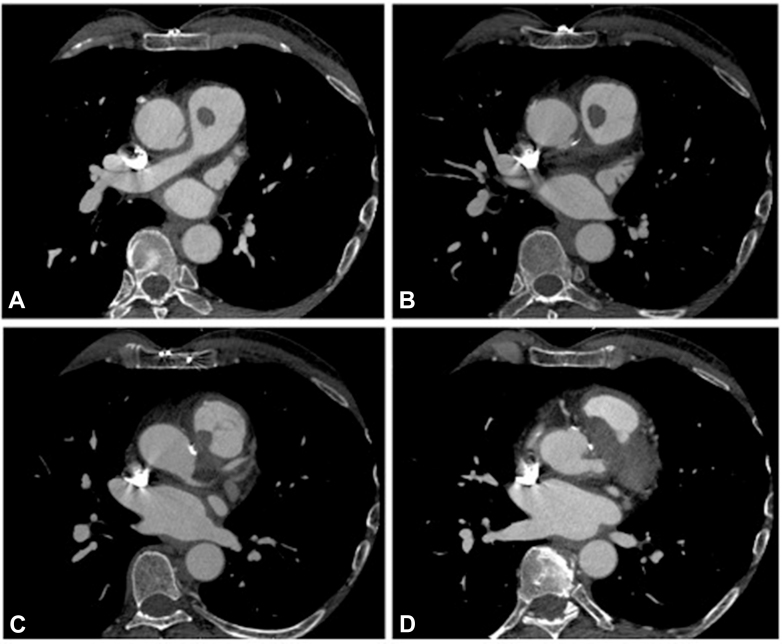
Craniocaudal sequence of 4 (A-D) axial contrast enhanced computed tomograms showing the perivalvular abscess between the great vessel roots and a 12 × 8-mm mass attached to the right pulmonary valve cusp. Note the proximity of the left main coronary artery posterior to the infected area.
